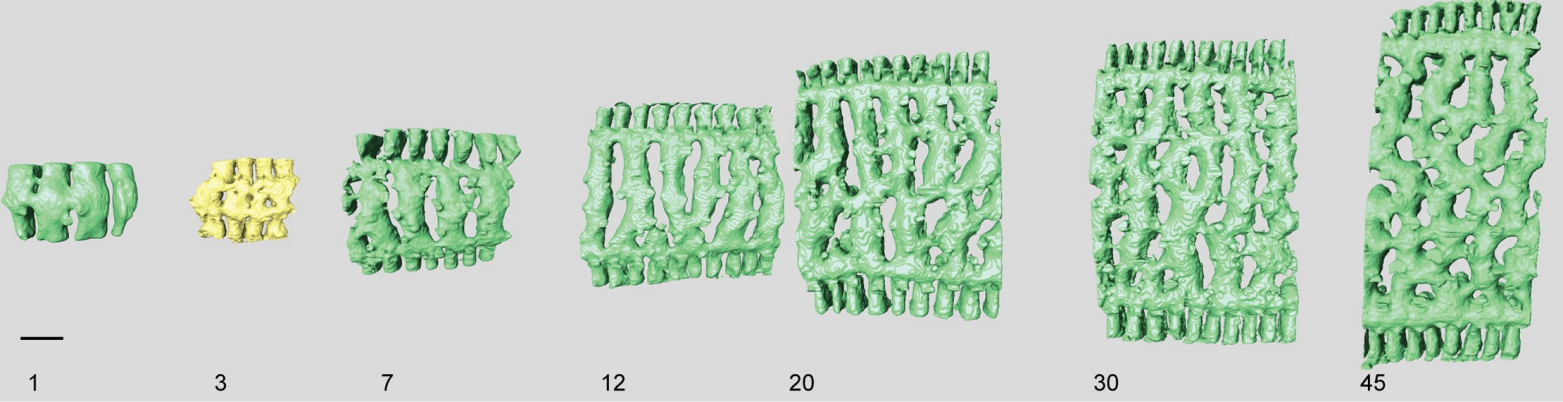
Micro-computed tomography of *Marginopora vertebralis* (RGM.1352105) with the shell removed, showing the interseptal space in chambers 1, 3, 7, 12, 20, 30, and 45. Note the increase in complexity and the frequent occurrence of annular connections in chambers 20, 30, and 45. Scale bar approximately 100 μm.
